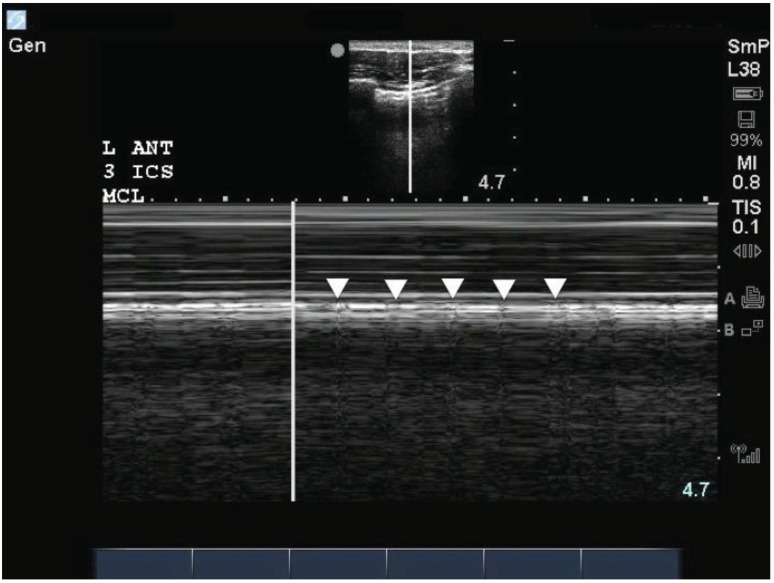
With M mode ultrasound, regular small movements (white arrowheads) can be seen under conditions with reduced lung sliding.
